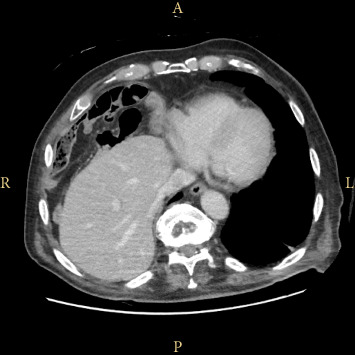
Axial CT scan showing Chilaiditi's sign; A (anterior), P (posterior), L (left), R (right).
